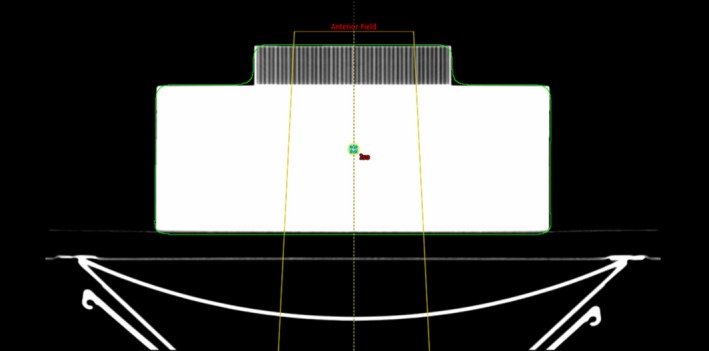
Experimental setup for attenuation measurements. A CT image with SolidWater^®^ and the representative cylinder are shown. Isocenter and the prescription point are located 5 cm depth in SolidWater^®^.
